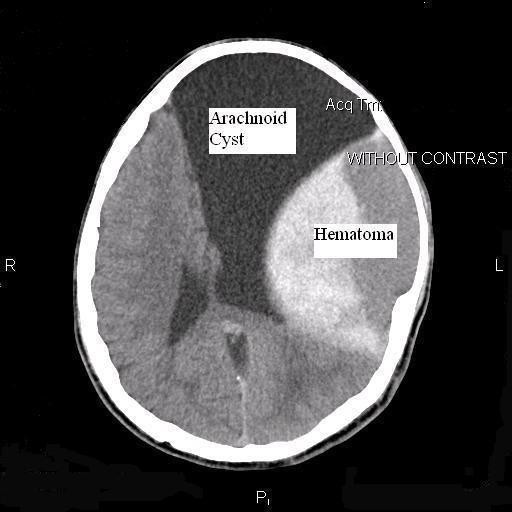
Brain CT scan of our patient. Shows the very large arachnoid cyst and extradural hematoma over the left frontoparietal convexity, with the bulged skull over the cyst.
